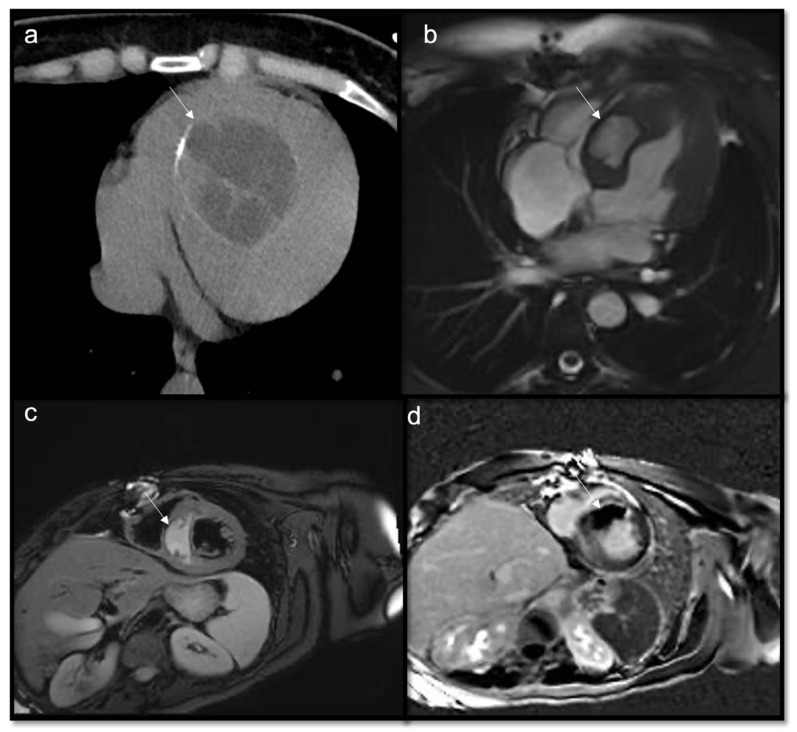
Cardiac hydatidosis (arrow) revealed in CT (a); CMR cine sequences showing a cyst within the septum (b), as confirmed in T2w Fat Sat (c), without late gadolinium enhancement (d). The standard treatment for cardiac hydatidosis is surgery as early as possible even if there is a high risk of the cyst rupturing inside the heart chamber.
